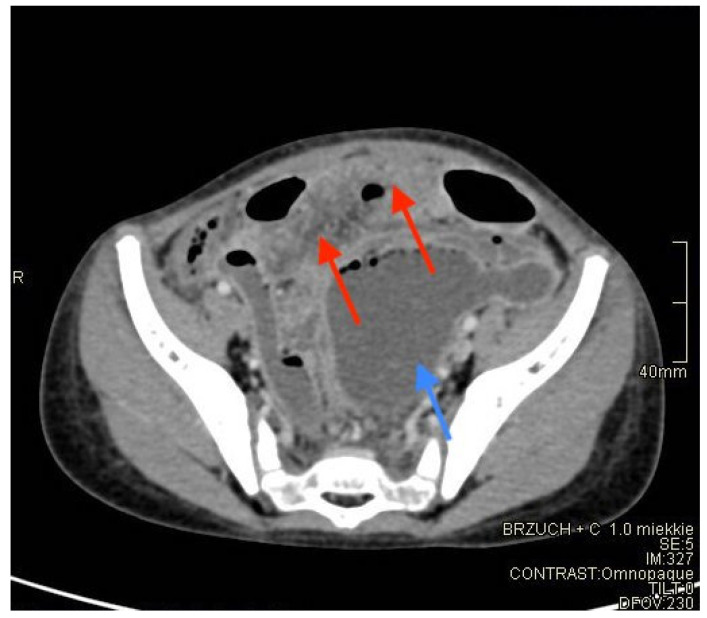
CT examination with contrast. Red arrow indicates mesenteric and omental fat stranding consistent with inflammatory infiltration and blue arrow—distended, fluid-filled lumen of the intestine.
